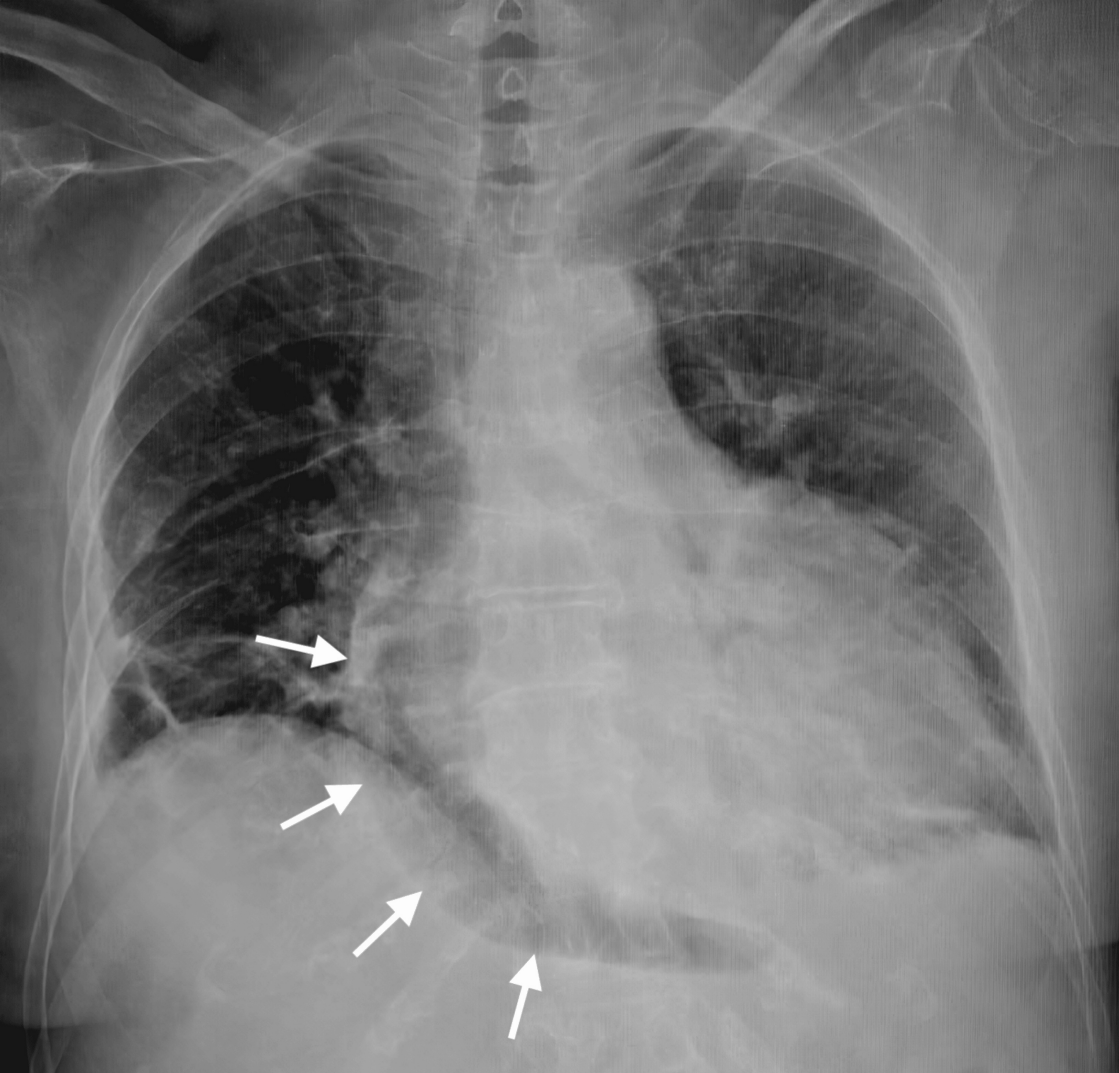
Anteroposterior (AP) chest radiograph. The image demonstrates a region of increased pericardial silhouette hypotransparency, an indirect radiographic sign suggestive of pericardial effusion.
